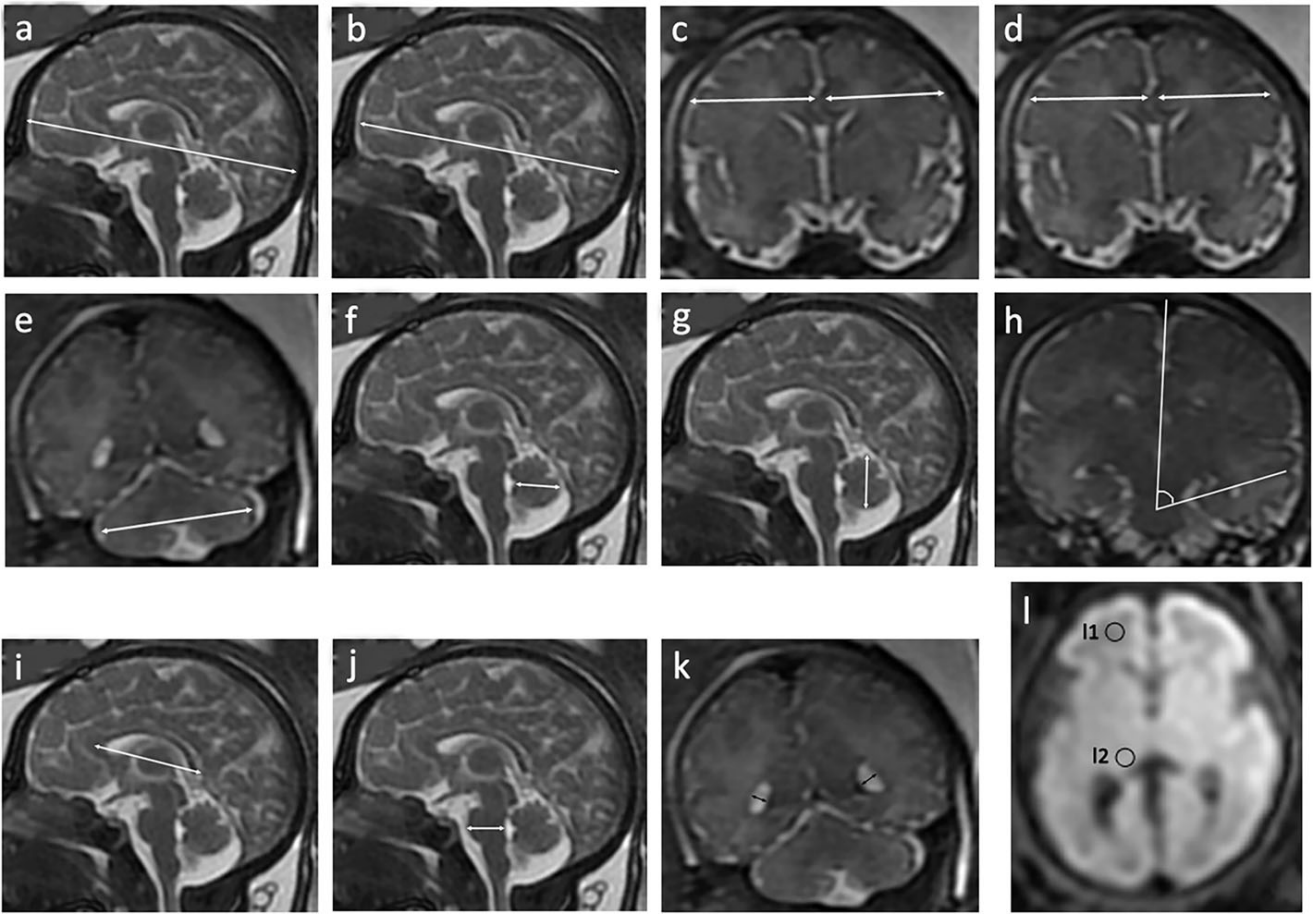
Magnetic resonance imaging slice showing measurement of: (a) bone fronto-occipital diameter; (b) cerebral fronto-occipital diameter; (c) left and right bone biparietal diameters; (d) left and right cerebral biparietal diameters; (e) transverse cerebellar diameter; (f) anteroposterior diameter of the vermis; (g) vermian height; (h) hippocampal infolding angle; (i) length of the corpus callosum; (j) anteroposterior diameter of the pons; (k) left and right lateral ventricles; and (l) apparent diffusion coefficient measures in frontal white matter (l1) and in basal ganglia (l2)
